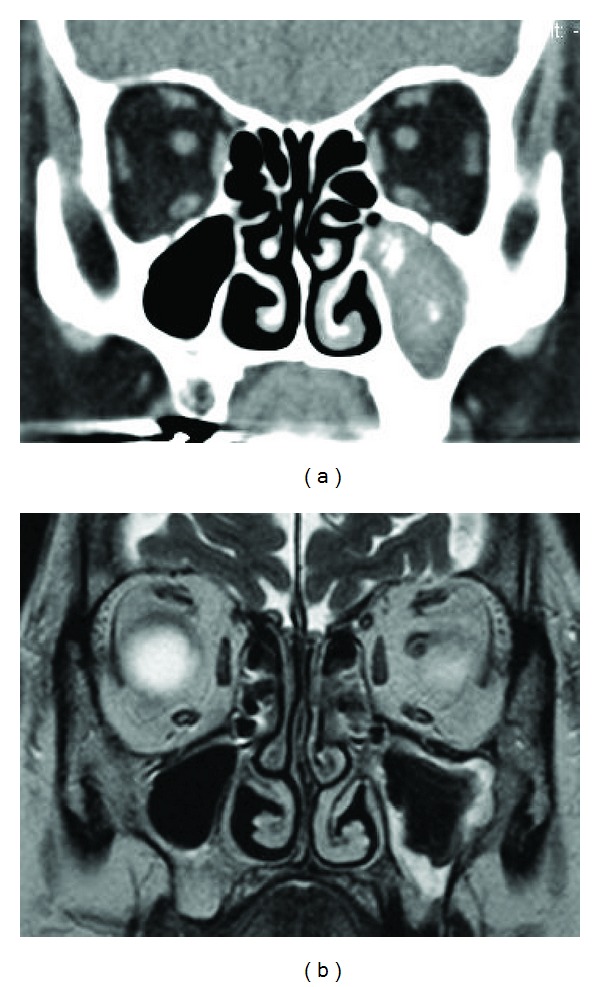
Typical neuroimaging findings of maxillary fungus ball. (a) Coronal CT scan with soft tissue density. Left maxillary sinus is completely filled with material. High density spots are seen. (b) Coronal T2-weighted MR image. Extremely low signal intensity to signal void indicates the presence of fungus ball. Peripheral high intensity area indicates edematous mucosa.
